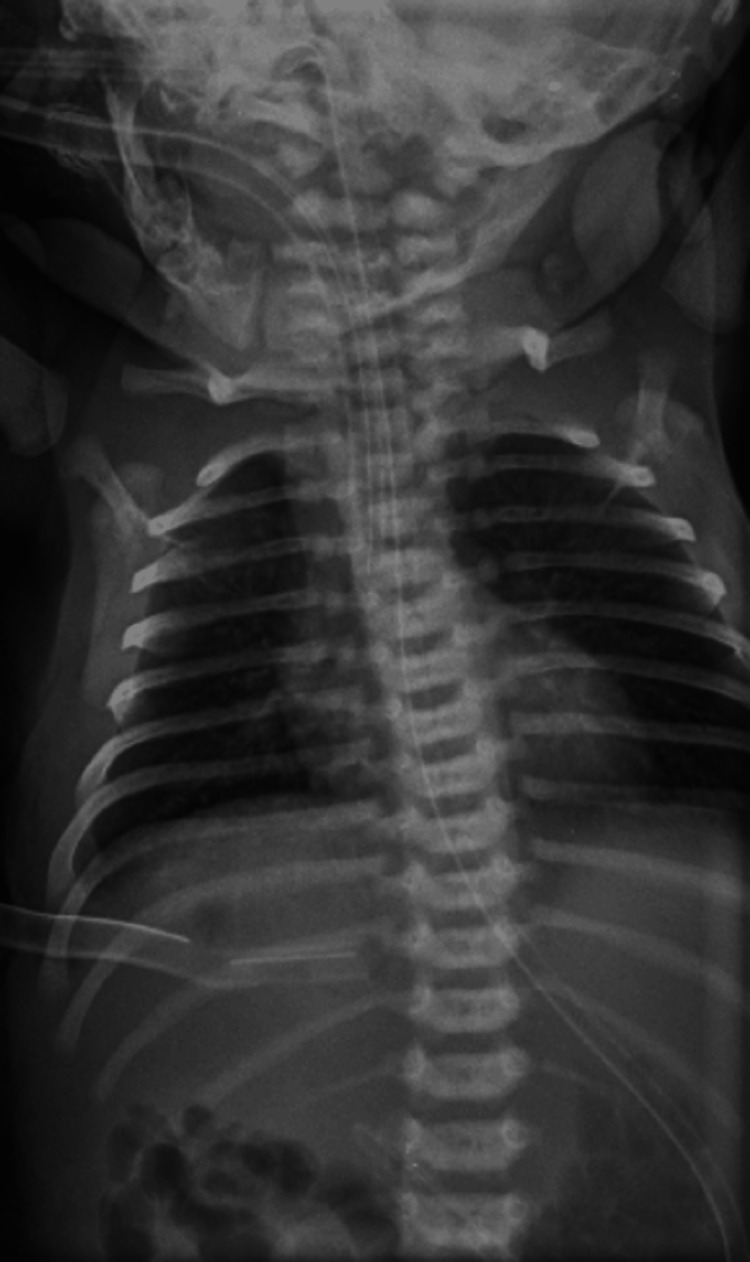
X-ray showing complete repair of the CDH. CDH: congenital diaphragmatic hernia.
